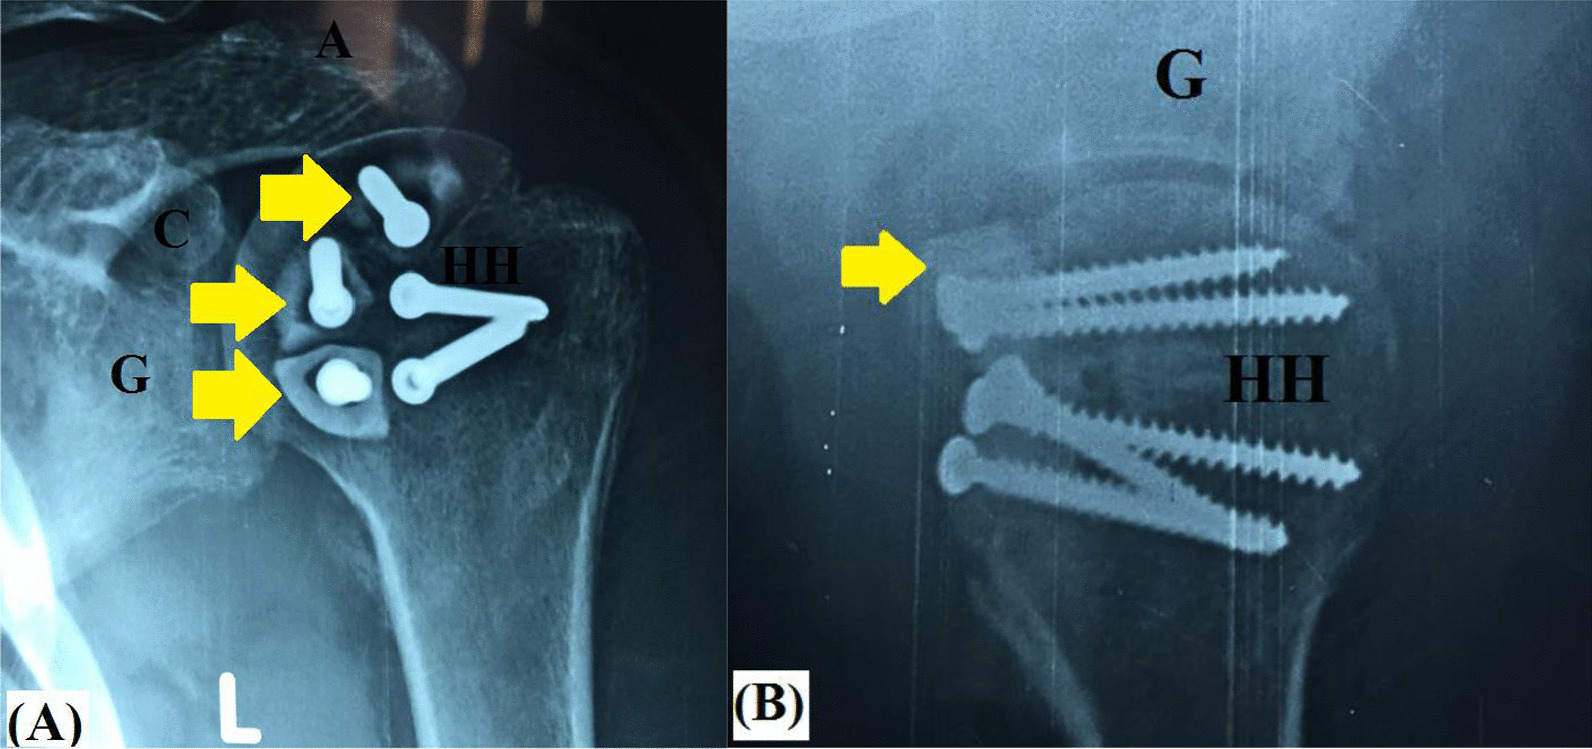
2.5-month postoperative ongoing healing of the (yellow arrow-marked) fibular graft into cancellous bed of reverse Hill-Sachs defect in left shoulder; A antero-posterior X-ray view; B axillary X-ray view; A, Acromion; C, Coracoid; G, Glenoid; HH, Humeral head
